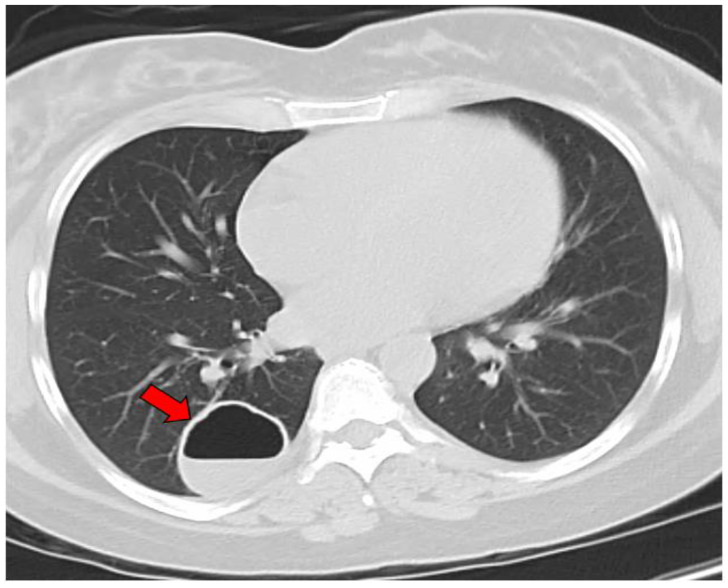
CT image of a single coccidioidal pulmonary cavity. The red arrow indicates a cavity.
